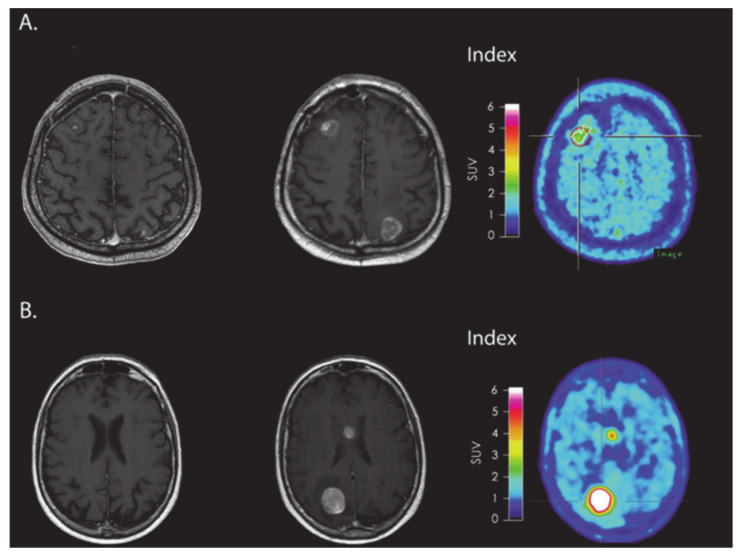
(A) MRI and FET-PET images of a patient with melanoma brain metastasis, diagnosed with PsP using immune-related response criteria (irRC) after receiving immune checkpoint inhibitor immunotherapy. The index MRI shows >25% increase in contrast-enhancing lesions located in frontal and occipital regions. Low metabolic tumor activity was observed on FET-PET images. (B) MRI and FET-PET images of a patient with melanoma brain metastasis, who was diagnosed with TP using irRC. The index MRI shows >25% increase in contrast-enhancing lesions located in the body of corpus callosum and occipital regions. A very high metabolic tumor activity was observed on FET-PET images. Reprinted with permission from ref. [109]. Copyright 2016 Oxford University Press.
